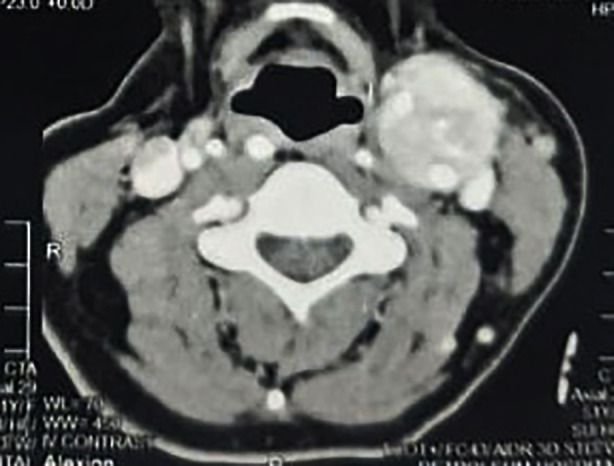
Axial CT Angiography Showing Carotid Body Tumour with Vascular Splaying.
